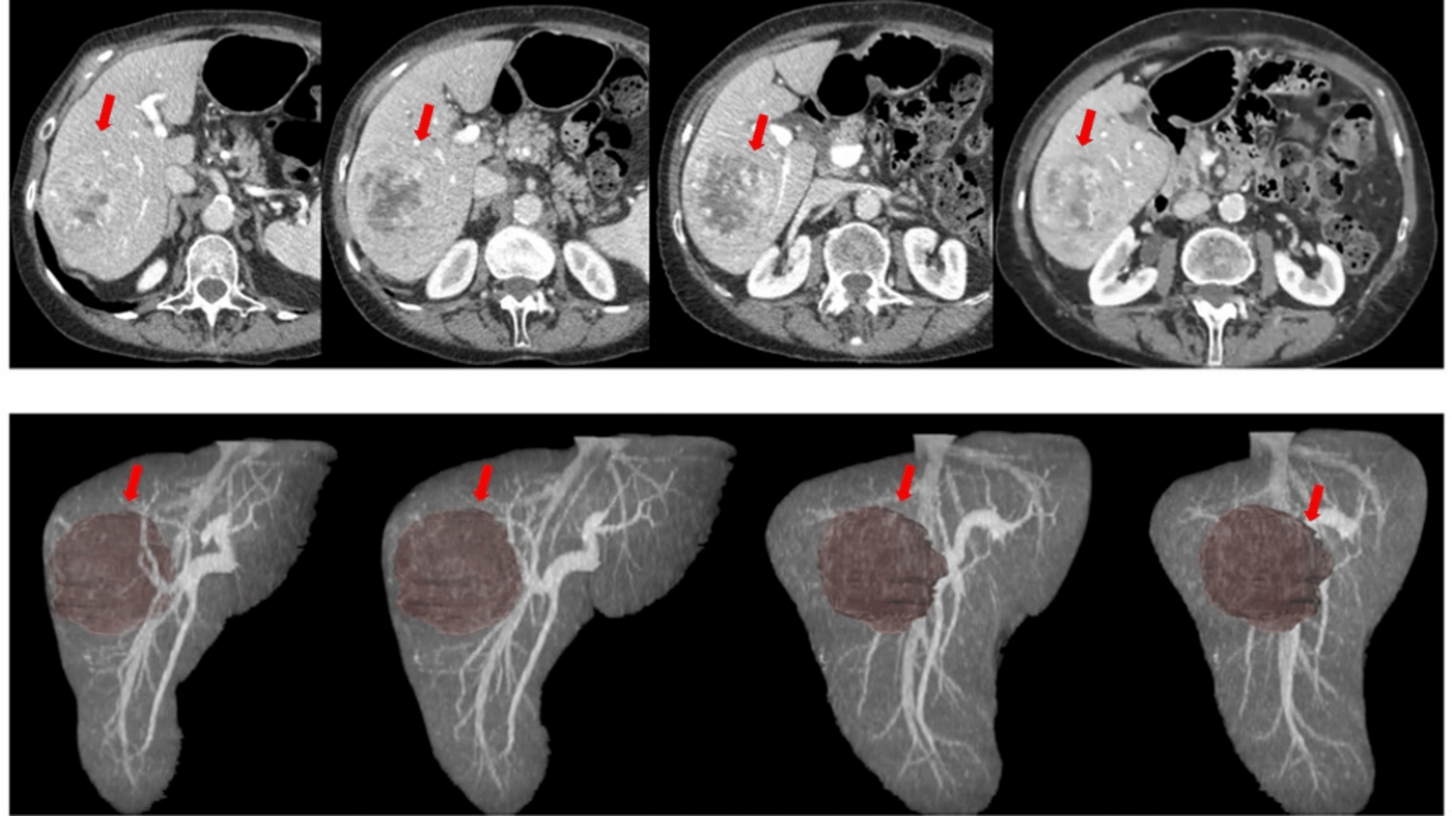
2D and 3D visualization of the liver 76-year-old male patient with a singular liver tumor in the right liver lobe. 2D axial abdominal CT images (top row) and corresponding semiautomated 3D reconstructions of the same patient (bottom row).
